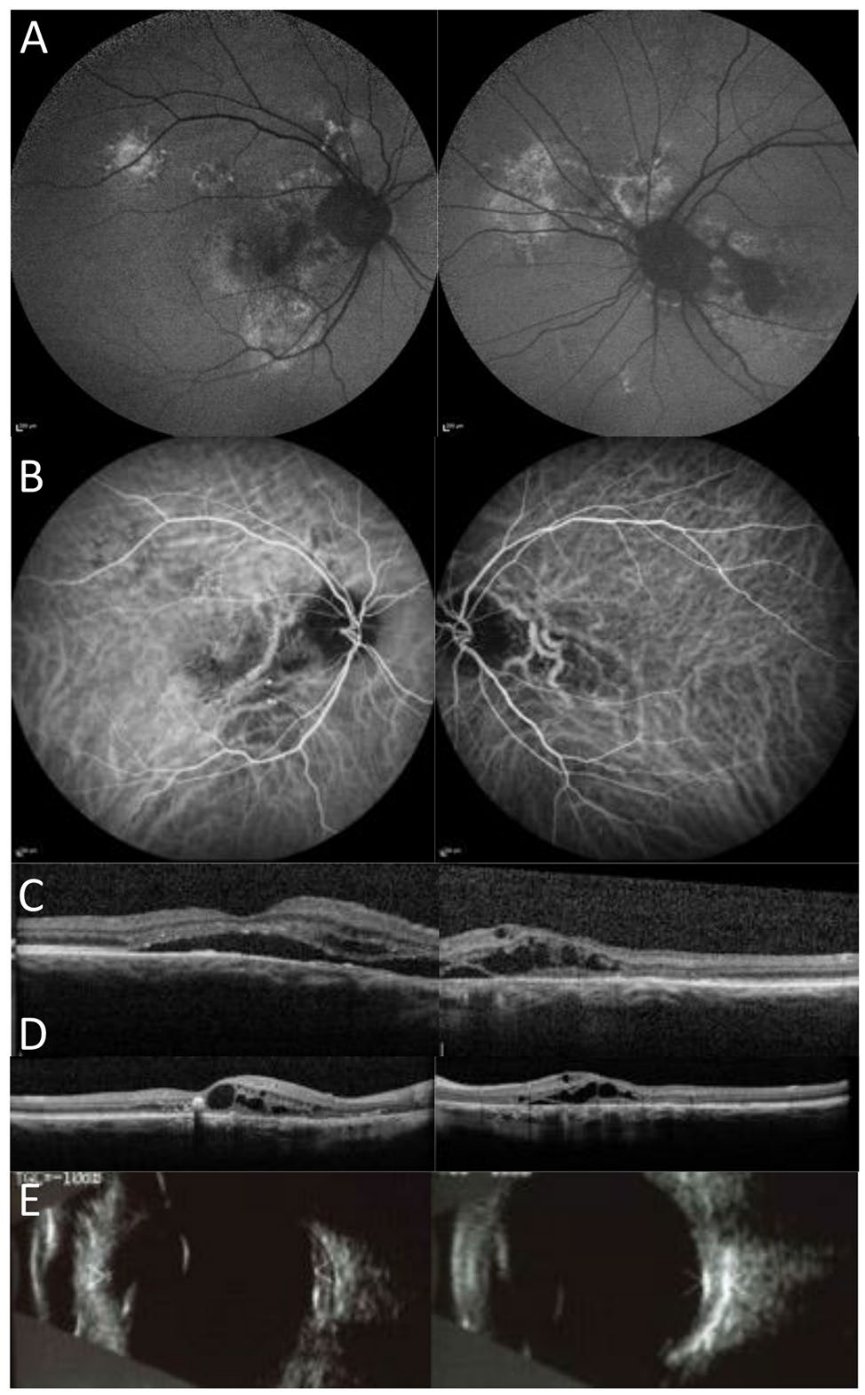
Multi-modal imaging for case 3 A) Fundus autofluorescence (FAF) imaging showing features of current and previous episodes of CSC in both eyes (B) ICGA imaging demonstrating CRF in the right eye and polypoidal choroidopathy (C-D) OCT imaging at baseline (C) showing bilateral CSC and intraretinal fluid and follow up OCT imaging (D) improvement of the CSC but with remaining intra-retinal fluid (E) Ultrasound B scan images showing bilateral thickened ocular coats.
